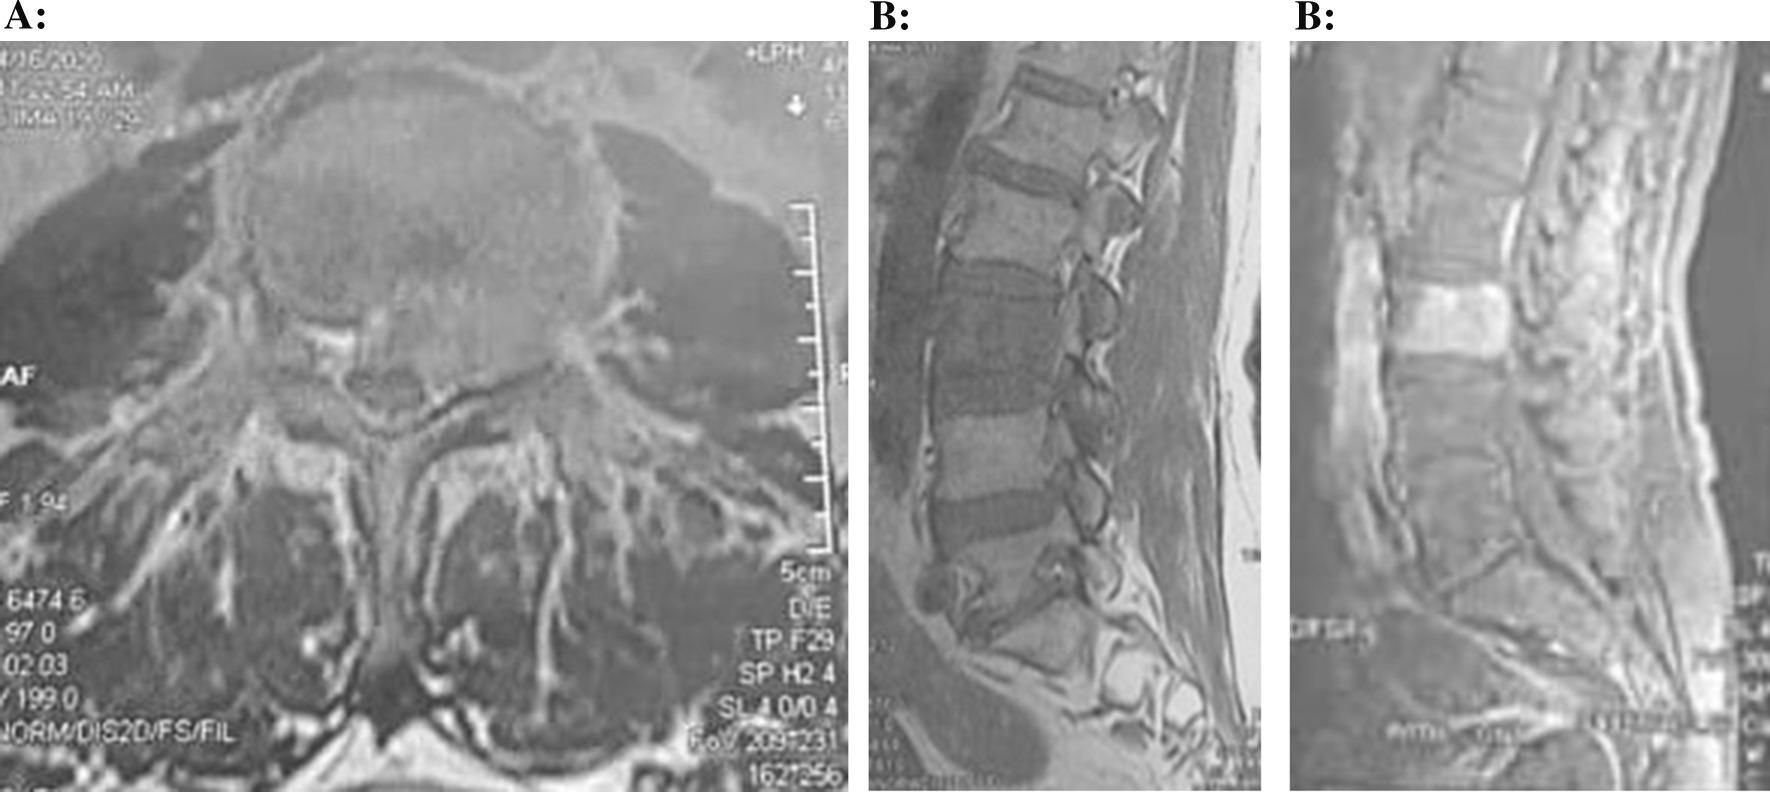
Axial (A) and sagittal (B) magnetic resonance images showing signal changes and cord compression on the L3 level
